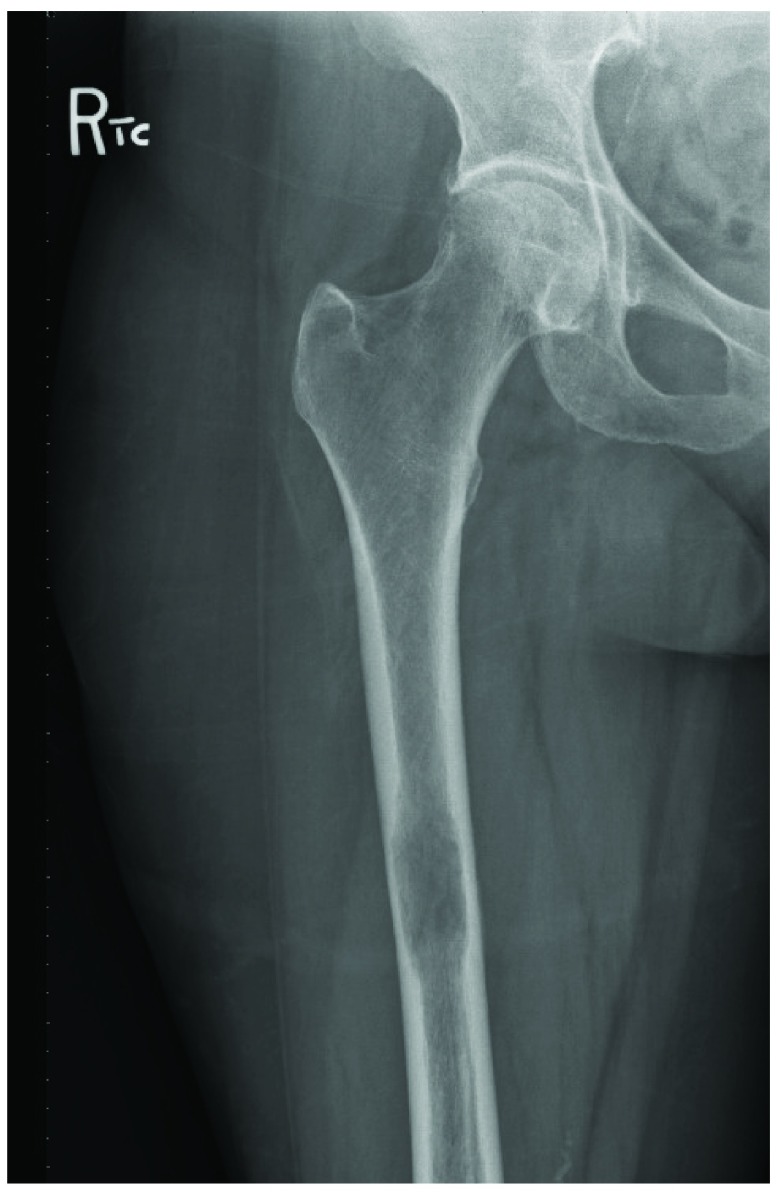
X-Ray right femur, AP view, demonstrates a large lucent lesion of the right femur with endosteal scalloping.
